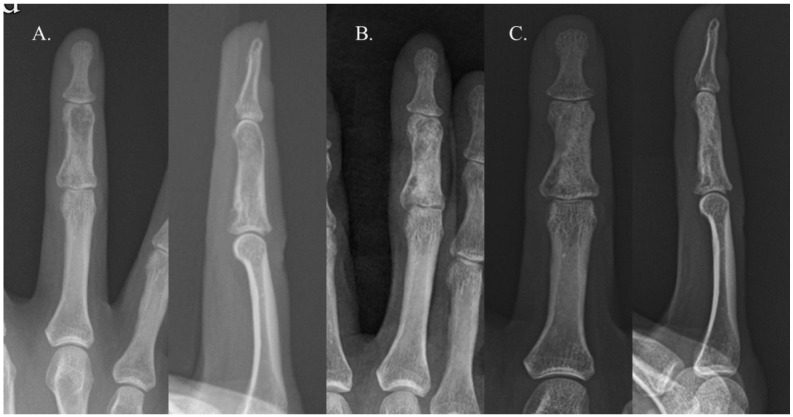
Simple radiographs of a patient with recurrence 6 years (A), immediately after surgery (B), and 1 year after surgery (C).
